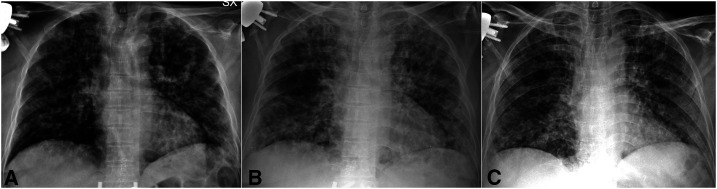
Plain X-rays. (A) Chest X-ray on admission showed bilateral pulmonary infiltrates. The distribution pattern is mainly peripheral. (B) Chest X-ray on day 14 showed worsening of the radiologic findings with peripheral and central distribution and bilateral evidence of areas of consolidation, particularly in the right lower field. (C) Chest X-ray on day 25 showed improvement of the radiological findings, particularly in the upper and middle fields, on both sides.
